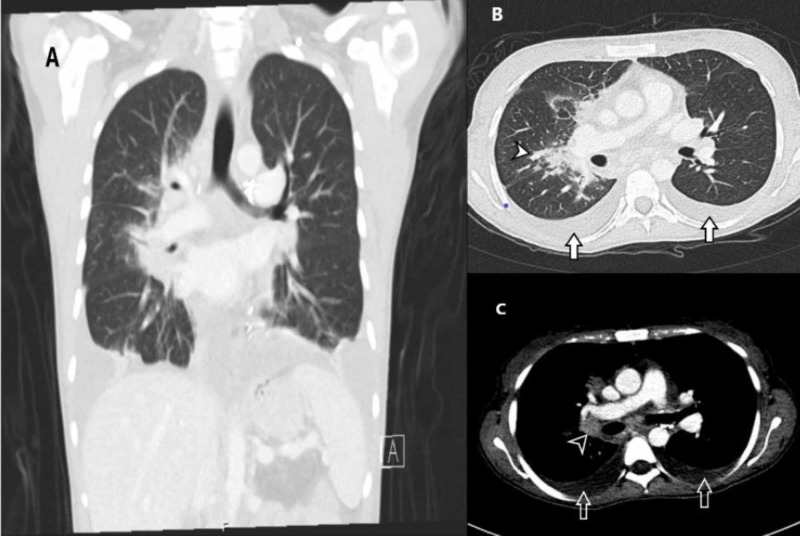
(A) Coronal CT image showing bilateral lower air space and perihilar opacity. (B) Bilateral pleural effusion (Arrows) and right perihilar opacity (Arrowhead). (C) Enlarged enhancing right hilar lymph node (Arrowhead) and bilateral pleural effusion (Arrows).
